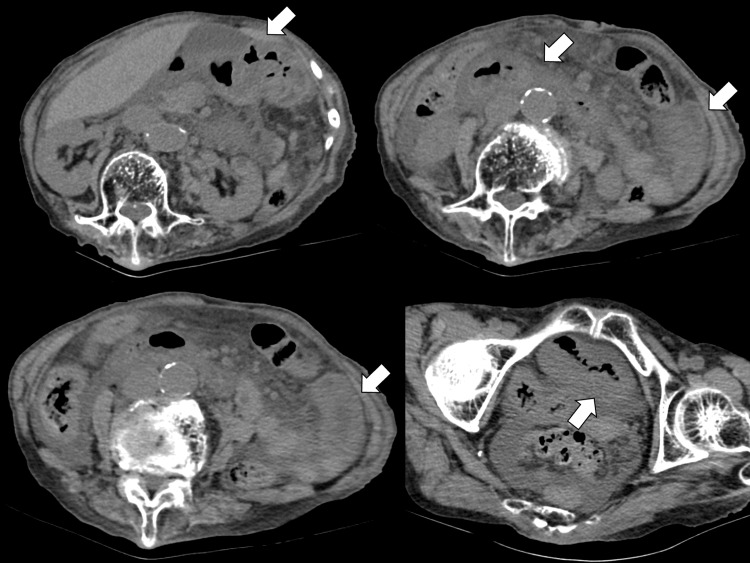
Computed tomography images of the abdomen The computed tomography shows the edematous walls of the stomach, duodenum, and small intestine with skipped lesions (white arrows)
